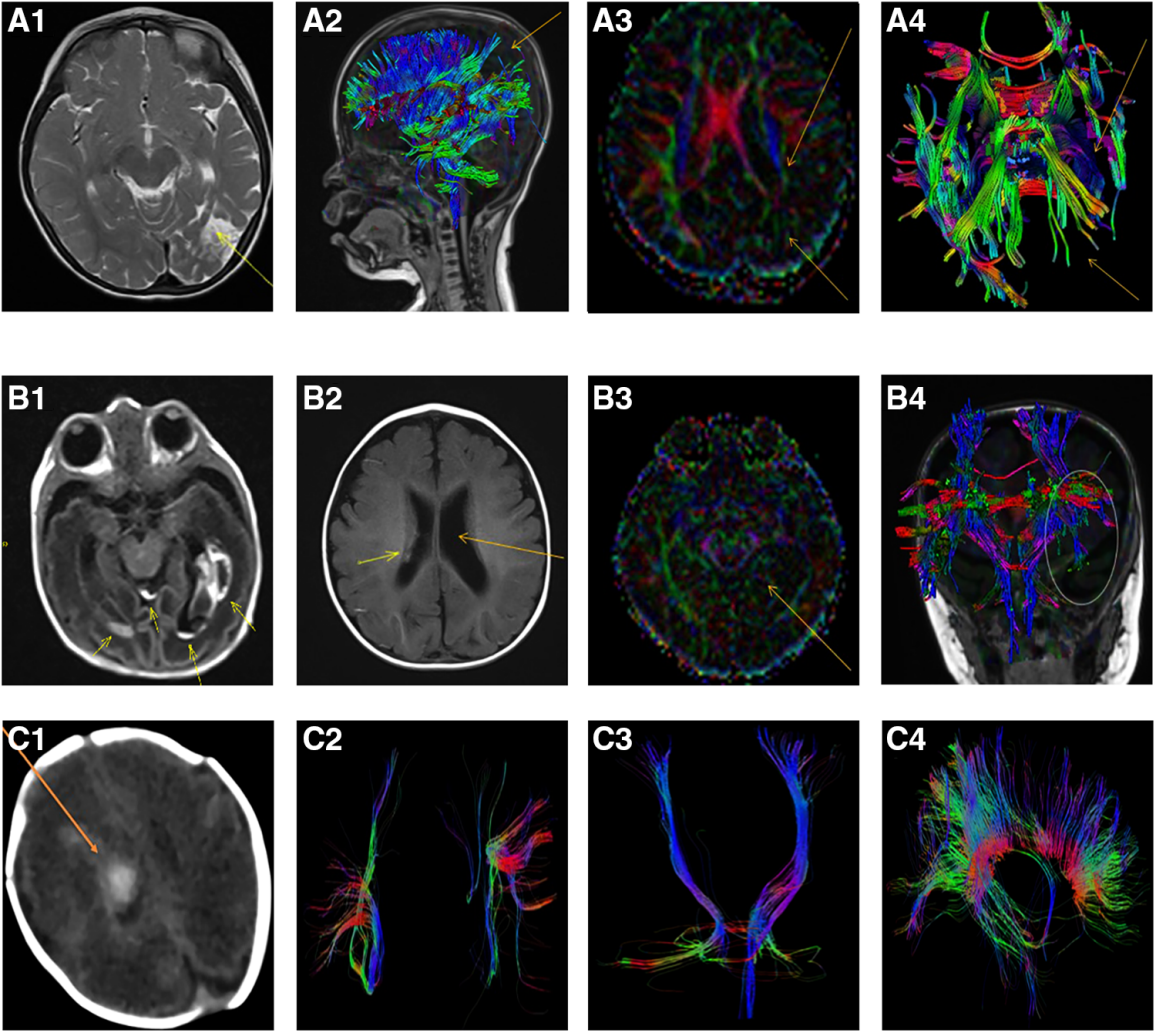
Representative lesions and the losses of nerve fiber bundles from MRI scan and DKI tractography in extremely preterm infants with severe intraventricular hemorrhage. (A1-A4): The loss of nerve fiber bundles in DKI tractography at 36 months after intraventricular hemorrhage of an extremely preterm infants with a gestation age of 27 weeks. (A1): Axial T2-weighted image showed hyperintense in the left temporal and occipital lobes which indicated the formation of softening lesions (arrow); (A2): Sagittal view, DKI tractography showed the loss of nerve fiber bundles in parietal and occipital lobes (arrow); (A3): The decrease and loss of signals of fractional anisotropy (FA) in left hemisphere with DKI scan, especially in the left temporal and occipital lobes (arrow); (A4): Axial DKI tractography showed the loss of nerve fiber bundles in the left temporal and occipital lobes (arrow); (B1-B4): An extremely preterm infants with a gestation age of 27 weeks. (B1): Axial T1-weighted image showed intraventricular hemorrhage and germinal matrix hemorrhage at birth (arrow); (B2): Axial T1-weighted image showed reduced hemorrhagic lesion in the right lateral ventricle and post-hemorrhagic ventricular dilatation of the left lateral ventricle at 6 months old (arrow); (B3): The differential signals of FA between the left and right hemisphere with DKI scan at 6 months old (arrow); (B4): Coronal DKI tractography showed the breakage and loss of nerve fiber bundles in posterior horn of the left lateral ventricle (ellipse); (C1-C4): An extremely preterm infants with a gestation age of 28 weeks. (C1): CT scan showed intraventricular hemorrhage at 12 days after birth (arrow); (C2): DKI tractography showed the right superior longitudinal fasciculus (SLF) became thin and sparse compared with the left SLF at 1 year of corrected age; (C3): DKI tractography showed the right corticospinal tract (CST) became thin and sparse compared with the left CST at 1 year of corrected age; (C4): Corpus callosum was showed by DKI tractography at 1 year of corrected age (Sagittal view).
